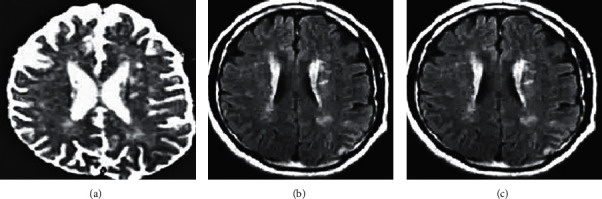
MRI image of a male stroke patient. (a) The diffusion limitation of the left frontal parietal cortex; (b) the diffusion limitation of the subcortical white matter; (c) the edema.
